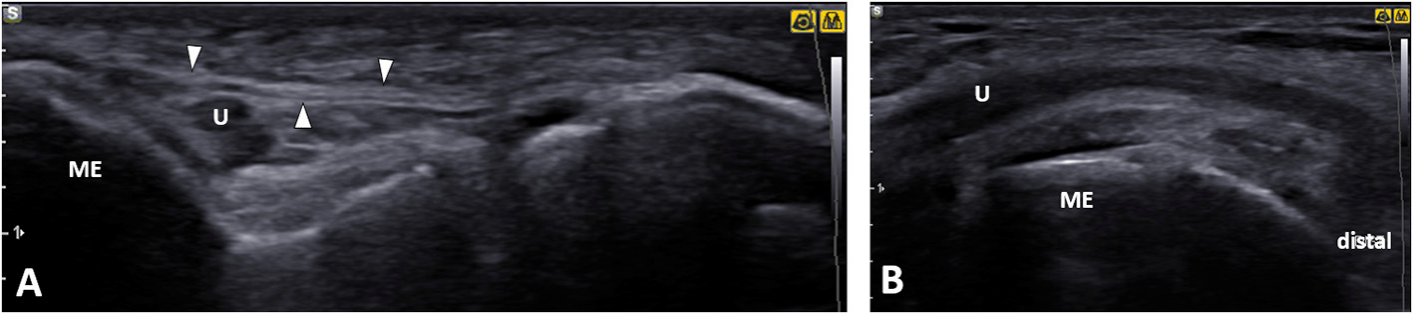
Normal ultrasound images of the ulnar nerve. (A) Short axis. (B) Long axis. U, ulnar nerve; ME, medial epicondyle; arrowheads, retroepicondylar retinaculum.
